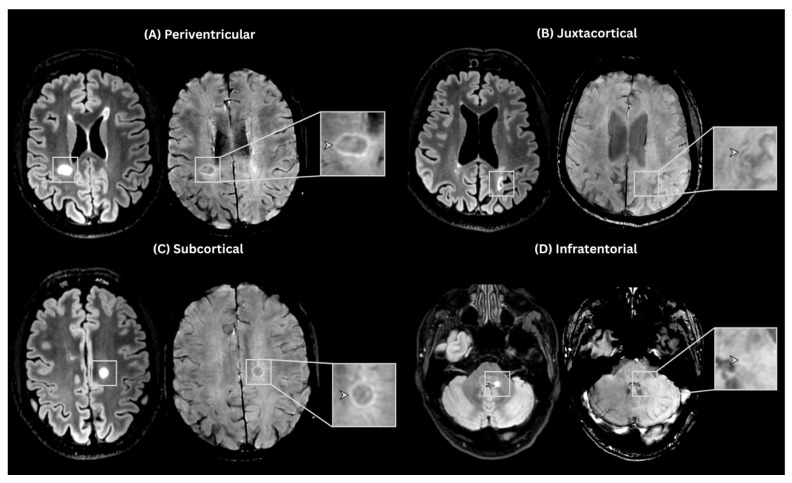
Examples of PRLs across different anatomical locations. Representative paired T2-FLAIR and SWAN images demonstrate PRLs. On FLAIR, lesions appear as hyperintense foci, while the corresponding SWAN images show a hypointense paramagnetic rim.
